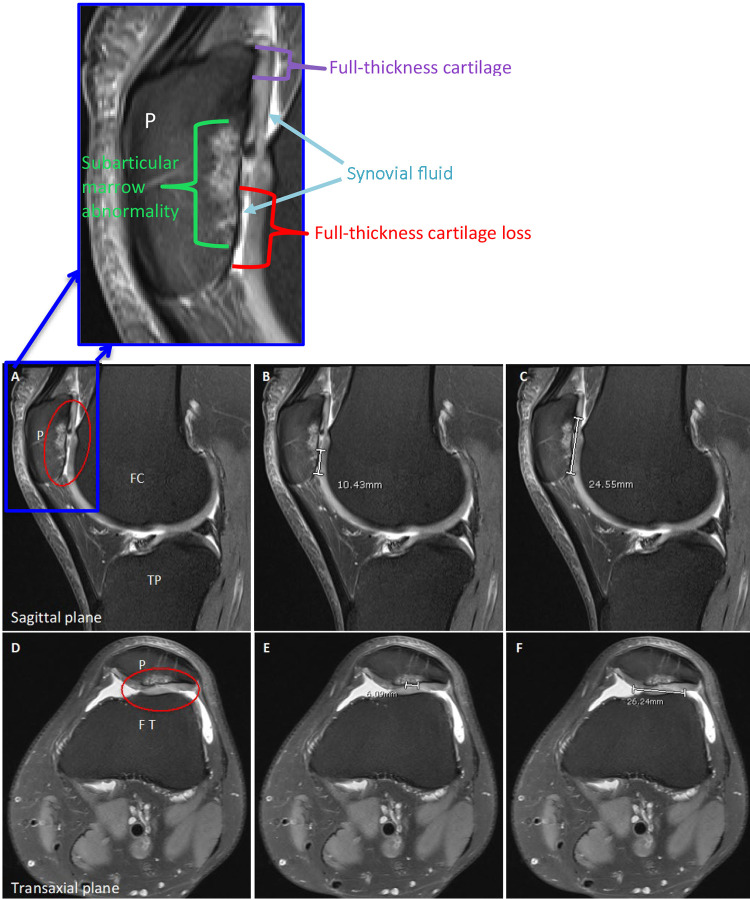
Preoperative 3-T proton density–weighted turbo spin-echo fat-suppressed magnetic resonance imaging scans of a patellar defect in the (A)-(C) sagittal and (D)-(F) transaxial planes. Inset shows the cartilage defect at a higher magnification, indicating abnormalities in the cartilage and bone. Defect areas outlined by a red oval highlight (A) the cartilage degeneration on the lateral patella with (D) matching preserved articular cartilage on the dysplastic femoral trochlea. (B, E) The full-thickness cartilage component of the patellar defect measured 10.4 mm × 6.1 mm (area = 0.6 cm^2^), whereas (C, F) the total predicted abnormal cartilage likely to be removed and treated with ACI was much larger, measuring 24.6 mm × 26.2 mm (area = 6.4 cm^2^; qualifying the patient for ACI^
